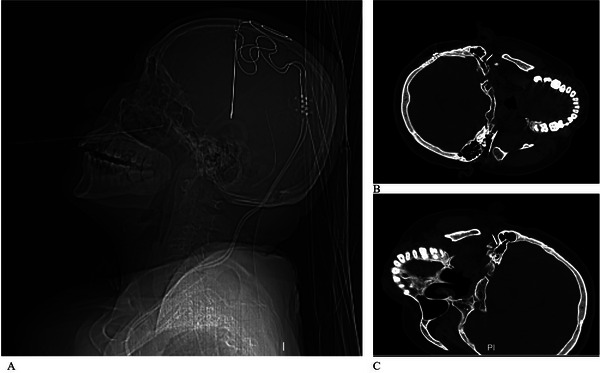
Intraoperative imaging of the repeat partial facial nerve RFA in Case 1. (A) CT 3D reconstruction visualizing the DBS electrodes and the radiofrequency needle trajectory toward the stylomastoid foramen. (B) CT‐guided needle placement at the right stylomastoid foramen. (C) CT‐guided needle placement at the left stylomastoid foramen.
